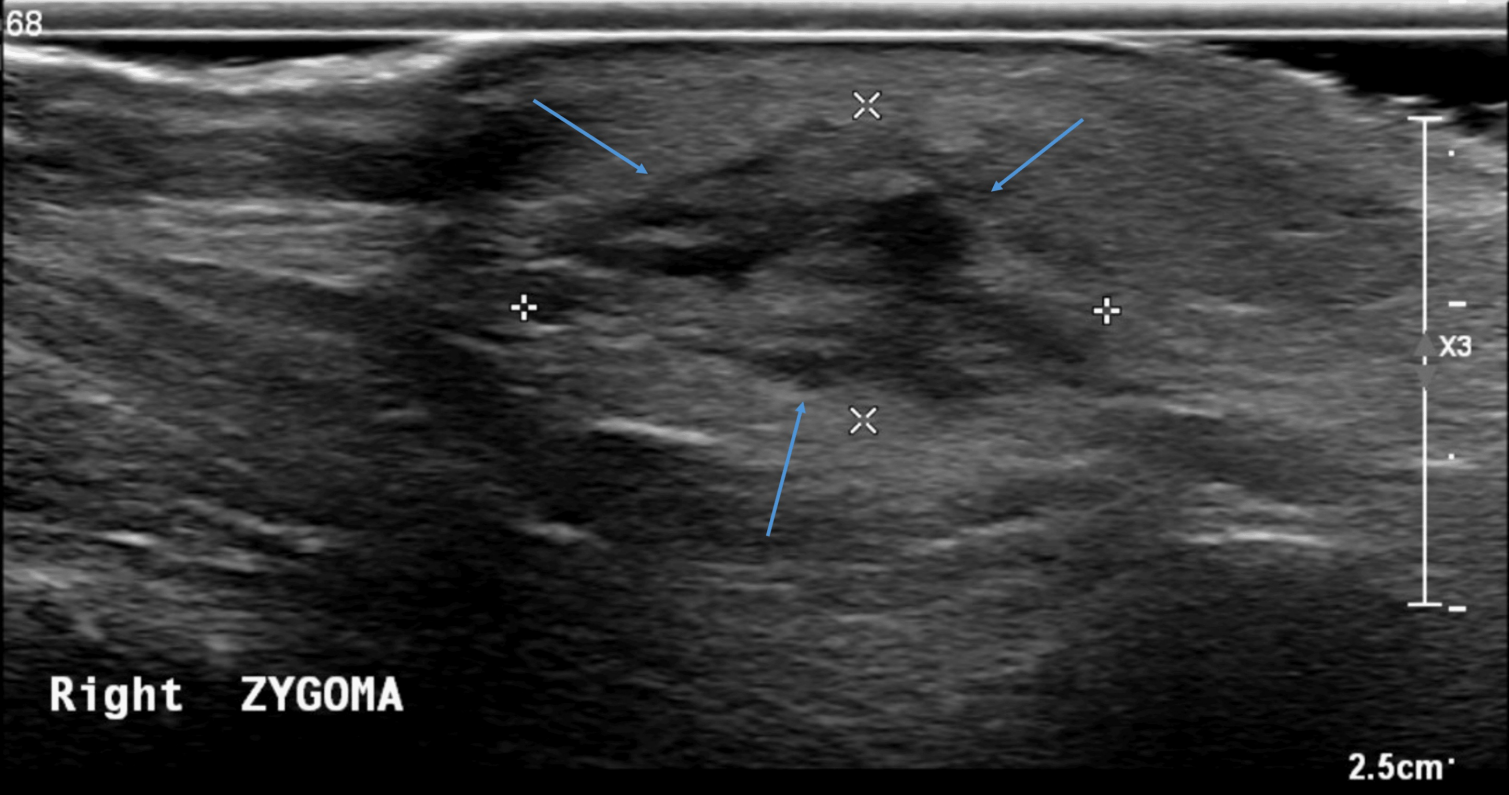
Ultrasonography of the lesion (May 2025). There is predominantly hypoechoic oval-shaped deep subcutaneous lesion against the right zygomatic arch (arrows), measuring approximately 19 x 10 x 23 mm. The lesion appears only minimally compressible, with no evidence of a foreign body or a tract to the skin surface.
